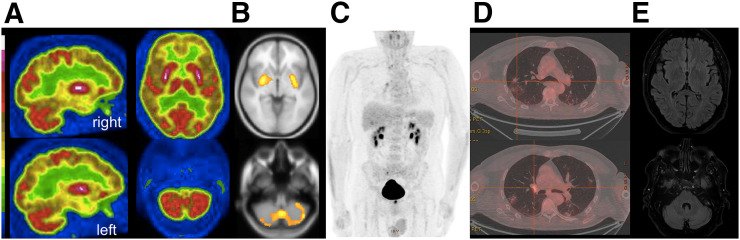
^18^F-FDG brain, whole-body PET, and brain MRI findings. (A and B) Brain ^18^F-FDG PET sagittal and axial slices showing diffuse cortical hypometabolism associated with putaminal and cerebellum hypermetabolism (A), confirmed by SPM comparison to 20 healthy elderly subjects (B) (*P* < 0.001, k > 600). (C and D) Whole-body ^18^F-FDG PET/CT showing slight lung and hilar lymph node hypermetabolism on maximum-intensity projection (C) and axial fused slices (D), matching pseudonodular retractile consolidation postinfectious findings on CT, without straightforward argument for neoplasia. (E) Axial slices from T2-weighted fluid-attenuated inversion recovery MRI of putamen and cerebellum showing no abnormalities.
